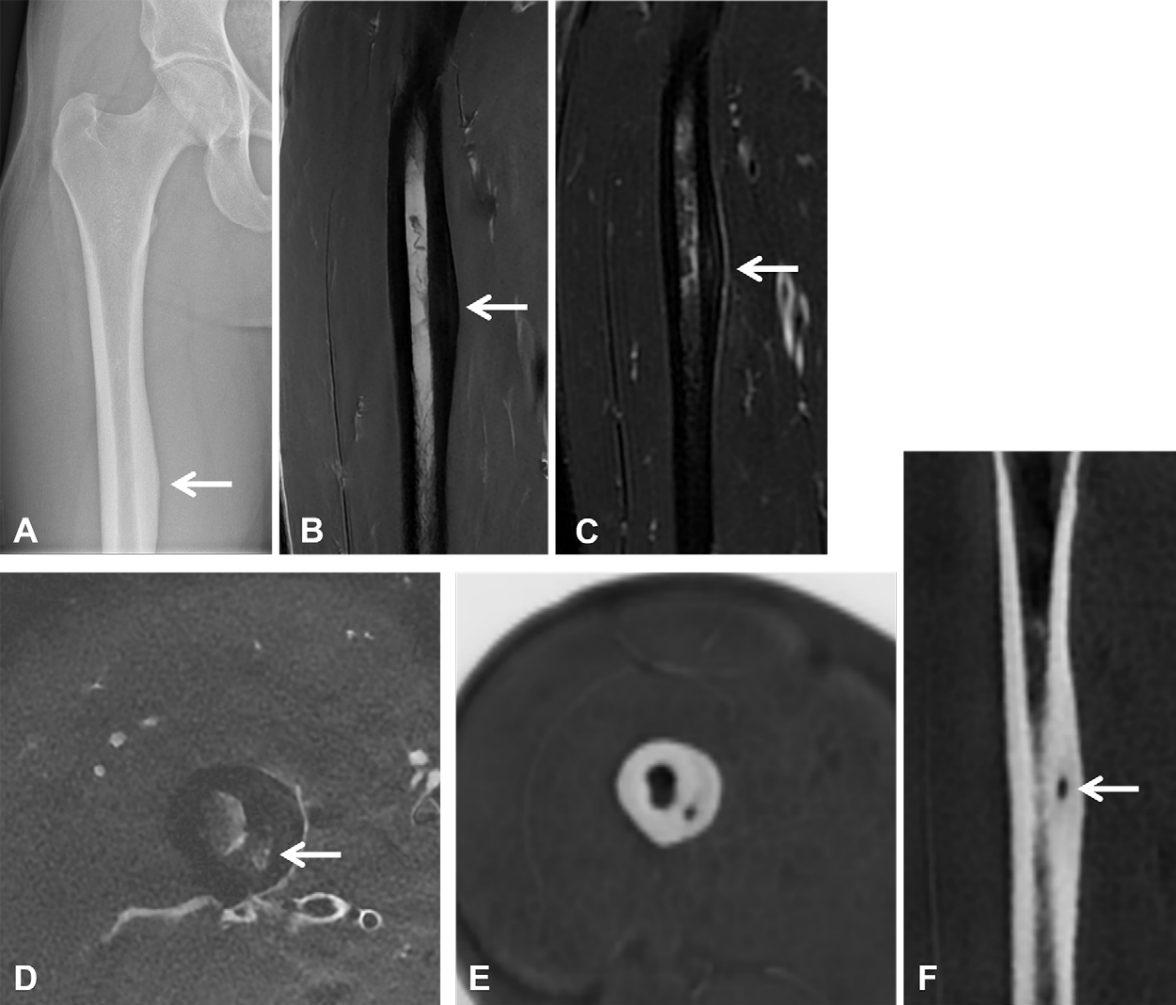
Osteoid osteoma of the right femoral diaphysis in a 23-year-old kickboxing male athlete. (A) Anteroposterior radiograph of the right femur demonstrates focal cortical thickening along the medial diaphysis (arrow). (B, C) Coronal T1-weighted and fat-suppressed T2-weighted MR images show medial cortical thickening with periosteal reaction (arrows). (D) Axial fat-suppressed T2-weighted MR image reveals a subtle central hyperintensity suggestive of a nidus (arrow). (E, F) Axial and coronal ZTE images clearly depict a small cortical nidus within the thickened cortex (arrows), confirming the diagnosis of osteoid osteoma.
